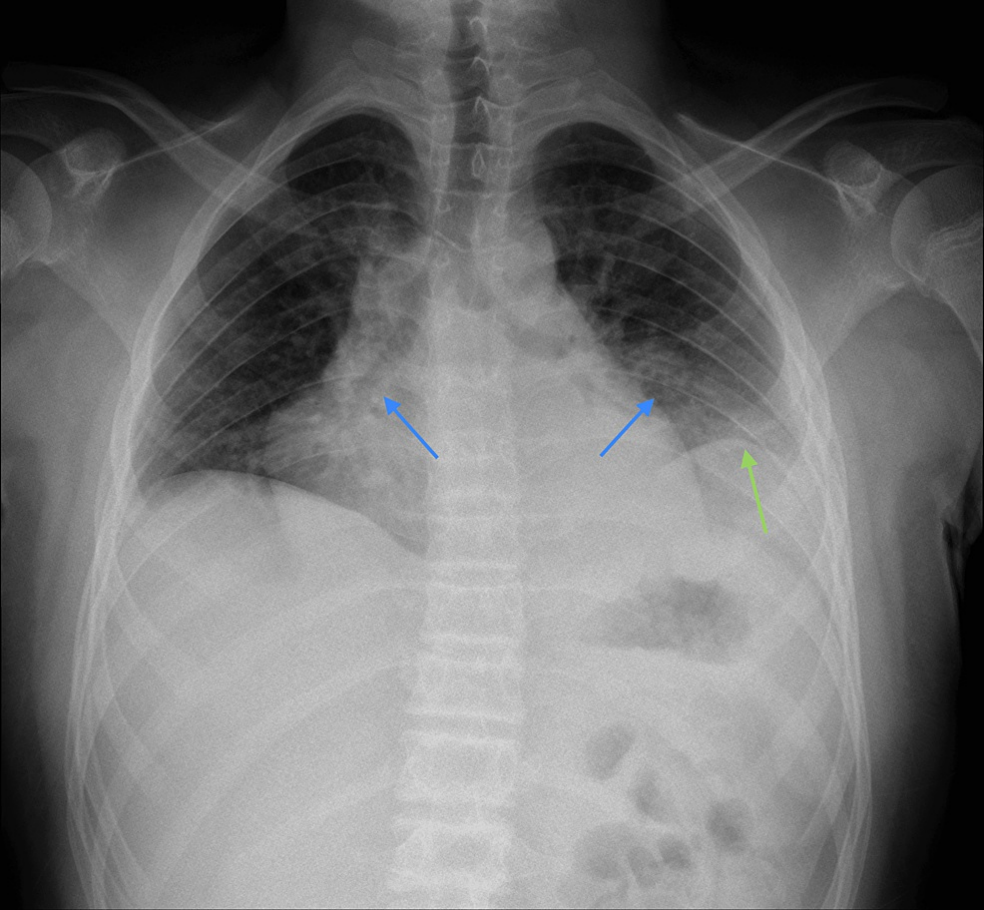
Preoperative X-ray Blue arrows: bilateral infiltrate, green arrow: left pleural effusion.
